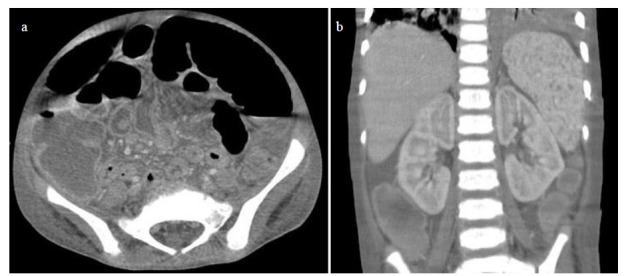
Abdominopelvic spiral computed tomography scan with contrast (with coronal reconstruction)
